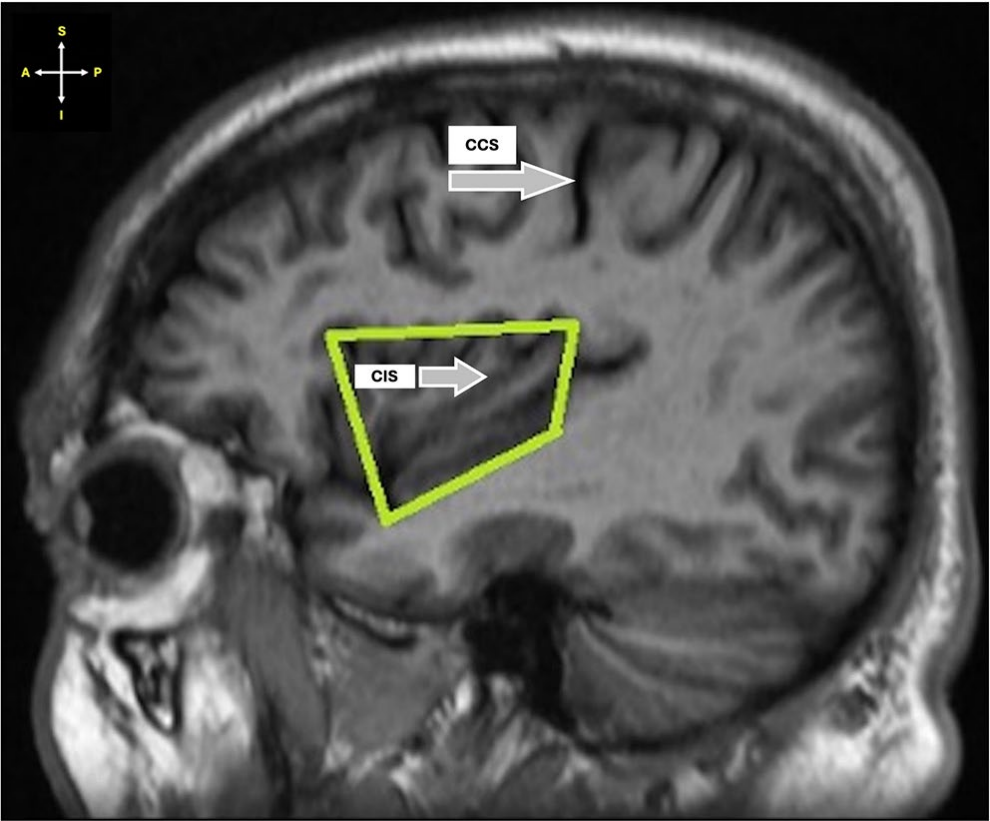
Sagittal section of MRI showing trapezoidal-shaped insula. *Key* CCS – Central cerebral sulcus; CIS – Central insular sulcus
